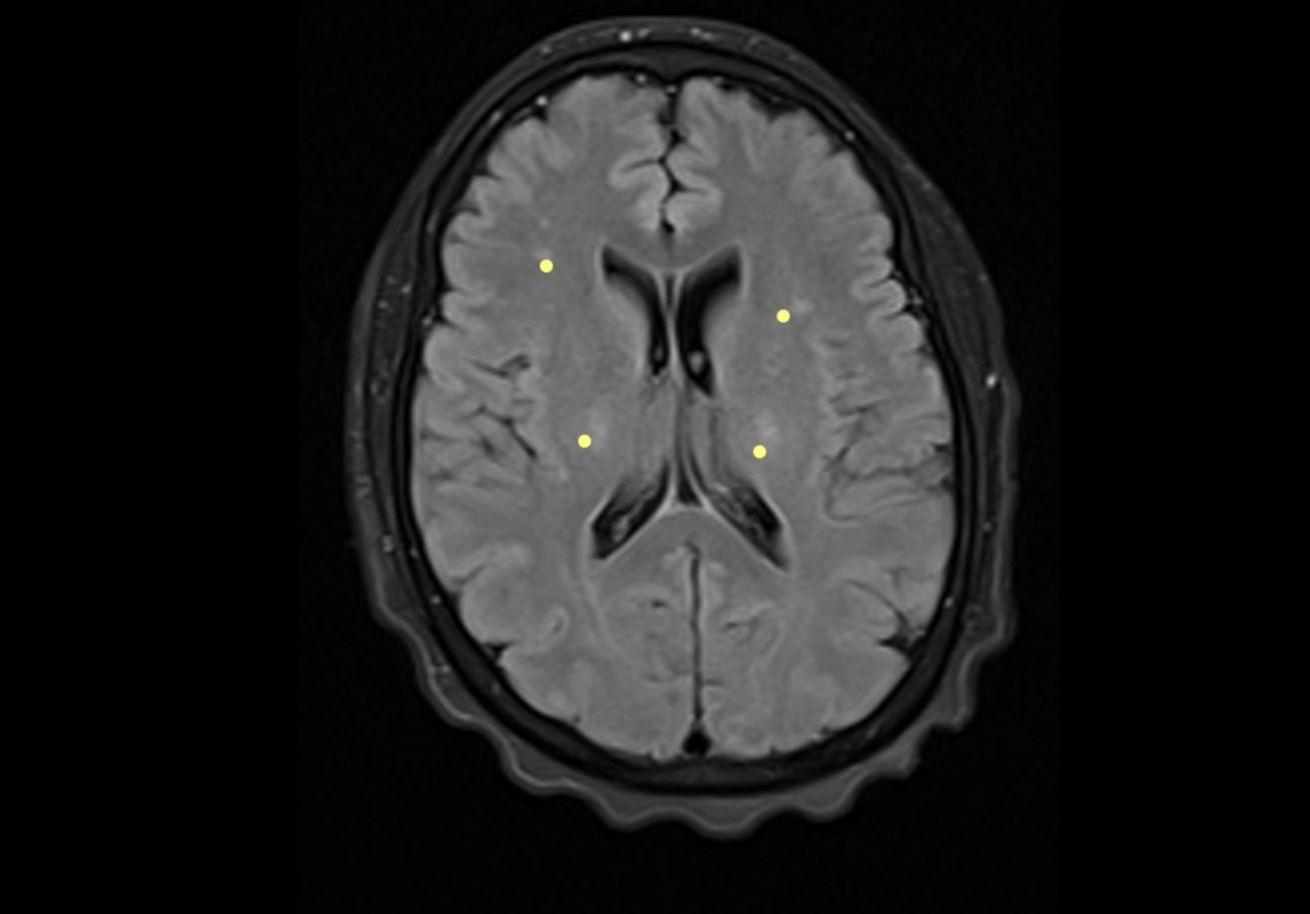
Brain MRI - view 5 FLAIR axial and T2-weighted coronal image. The image shows hyperintensity in the path of the corticospinal tract (yellow dots) MRI: magnetic resonance imaging; FLAIR: fluid attenuation inversion recovery
